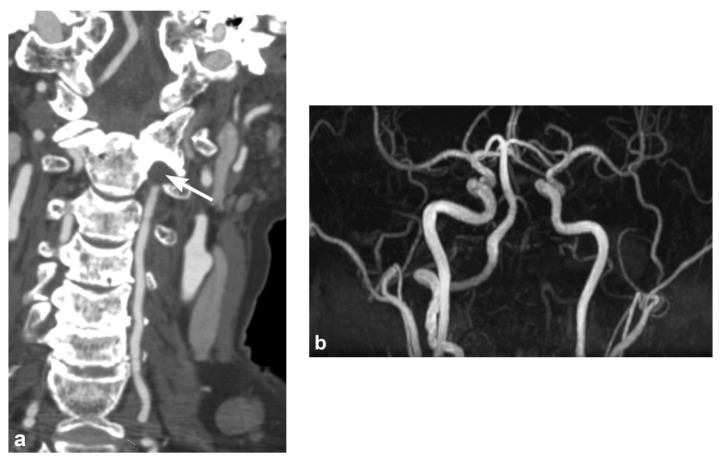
Left vertebral artery occlusion in a 77-year-old male patient involved in a motor vehicle accident. (a) Coronal multidetector CT angiographic image shows occlusion of the left vertebral artery with abrupt cut off. (b) Three-Dimensional Time-of-Flight MR Angiography of the Circle of Willis shows the long segment left vertebral artery occlusion extended to the intracranial segments.
